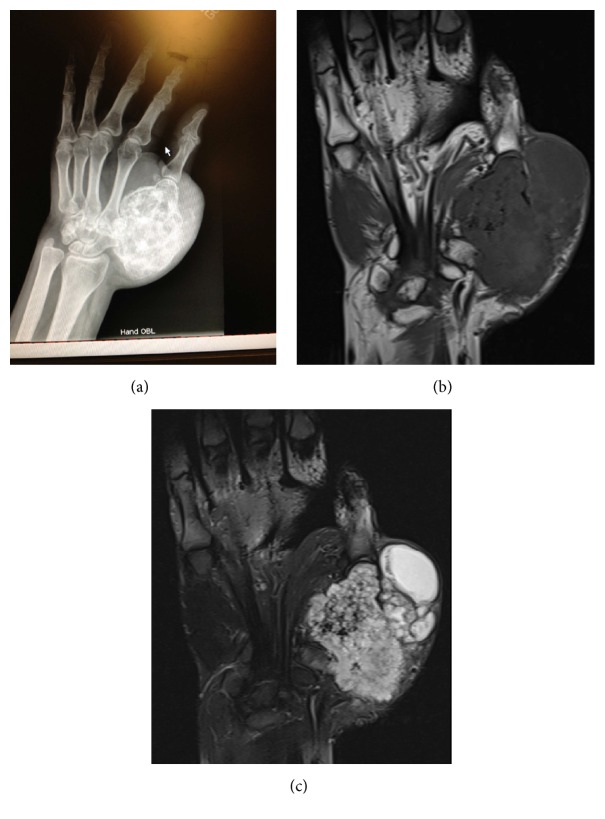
Preoperative imaging. (a) X-rays demonstrating a large, calcified tumor arising from the first metacarpal. (b) Coronal T1-weighted MRI of the left hand. (c) Coronal T2-weighted MRI of the left hand.
